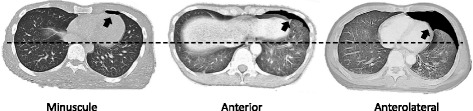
Occult pneumothorax size on CT scan classification by Wolfman et al. [15]. Minuscule pneumothorax is defined as a thin collection of air up to 1 cm thick in the greatest slice and seen on no more than 4 cm length. Anterior pneumothorax is categorized as a collection of pleural air more than 1 cm thick, located anteriorly, not extending to the mid-coronal line, and which may be seen on 4 cm or more length. Anterolateral pneumothorax is defined as pleural air extending to the mid-coronal line at least
